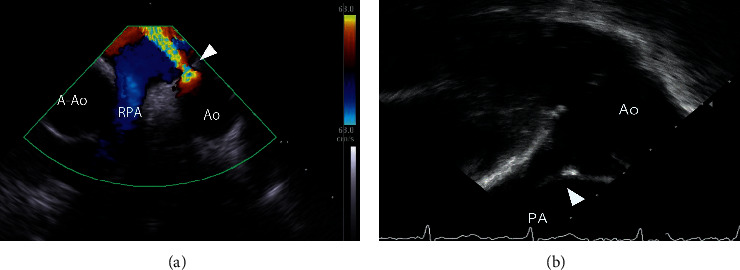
Representative image of MPA and LPA views of PDA using ICE. (a) The MPA view shows the aortic short axis view. (b) The LPA view shows the PDA long axis. A‐Ao, ascending aorta; Ao, aorta; ICE, intracardiac echocardiography; LPA, left pulmonary artery; MPA, main pulmonary artery; PA, pulmonary artery; PDA, patent ductus arteriosus.
